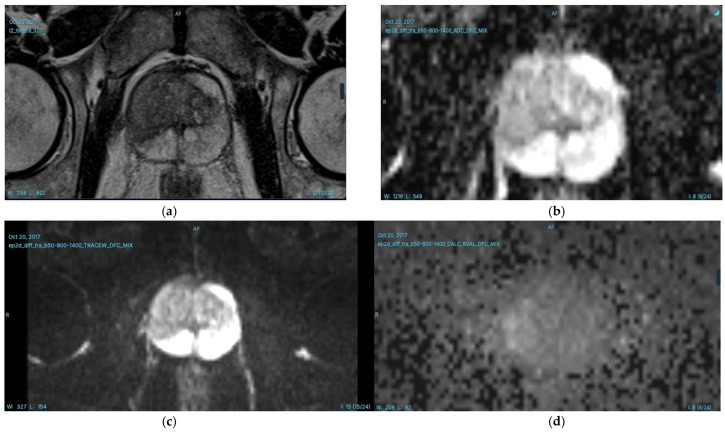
T2WI (a) ADC map (b) DWI) (c) and calculated *b*1500 DWI (*d*) images of a 63-year-old man with a serum prostate-specific antigen (PSA) level of 3.7 ng/mL. A focal hypointense prostate lesion is identified in the right peripheral zone (PZ), measuring 28 mm in diameter. The lesion is markedly hypointense on the ADC map and slightly hyperintense on high *b*-value images. According to PI-RADS v2.1 criteria, the lesion is classified as PI-RADS 3. Additionally, another PI-RADS 3 lesion is observed in the left lateral PZ, measuring 14 mm in diameter. This lesion appears focal hypointense on T2WI, markedly hypointense on the ADC map, and is indistinguishable on high *b*-value diffusion-weighted images.
